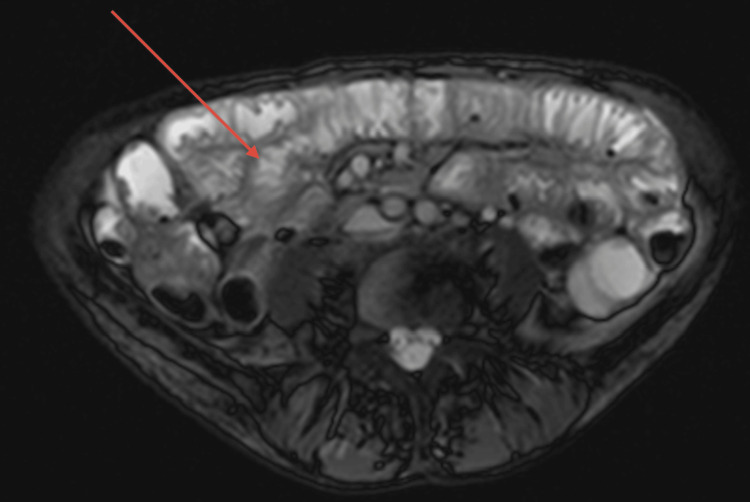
MRI small bowel showing thickening of the folds and jejunization of the ileal loops, which indicates a high likelihood of developing malignancy.
